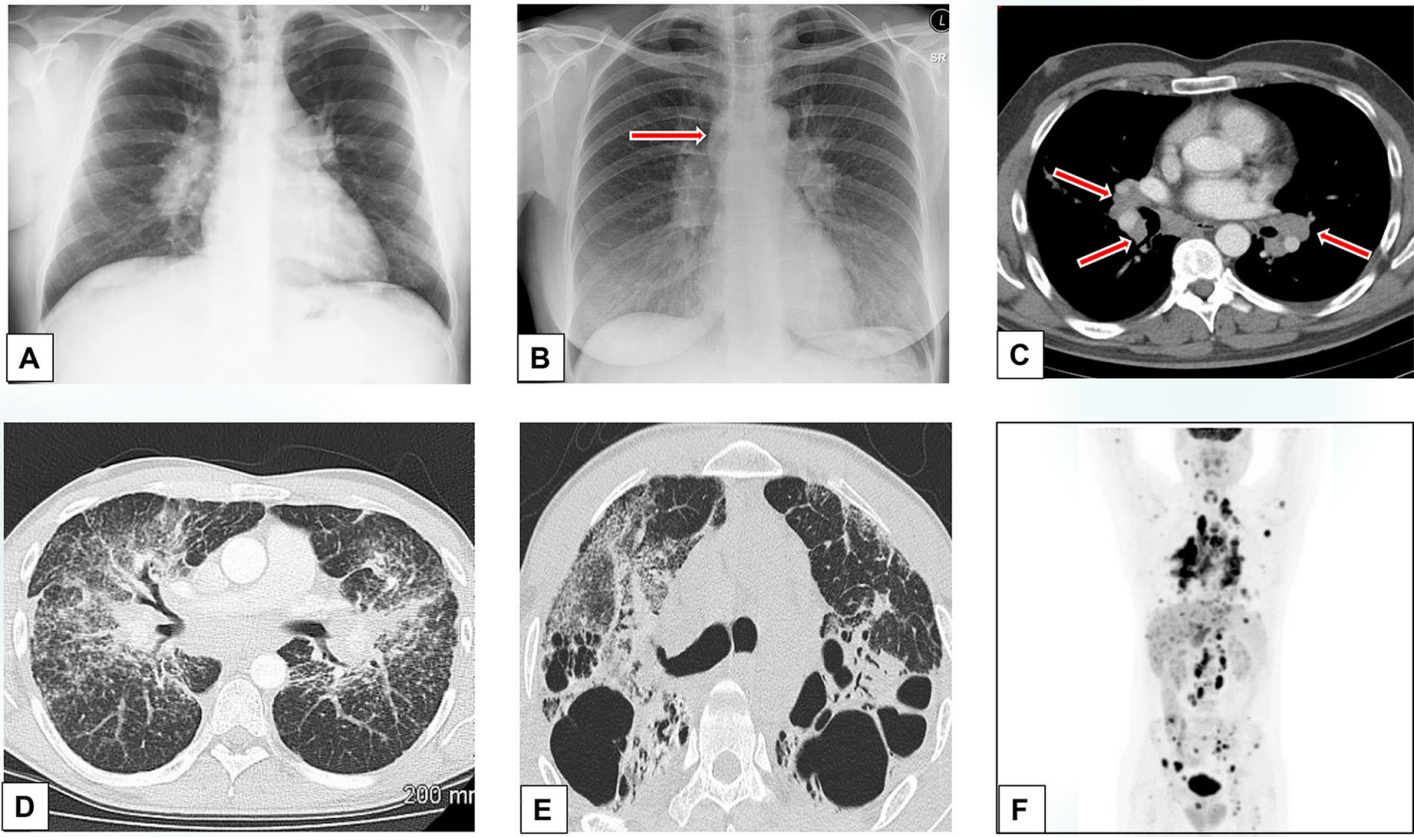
Radiographic patterns seen in the thorax of patients with systemic (including ophthalmic) sarcoidosis. a Bilateral hilar lymphadenopathy (BHL) with polycyclic outlines. b In another patient, BHL is associated with mediastinal enlargement along the right para-tracheal boundary (arrow). c Axial contrast computed tomography (CT) image shows typical BHL (arrows). Lymph nodes with a density lower than that of the vasa are disposed around the bronchi and pulmonary arteries. d Micronodules a few millimeters in diameter tend to form granulomatous conglomerates that surround the broncho-vascular peduncles. Lung involvement extends along the broncho-vascular axis, from the hila toward the periphery. Note the diffuse distribution in both lung fields of lung nodules with a typical peri-lymphatic distribution and thickening of the pleural surfaces. e Advanced-stage pulmonary sarcoidosis. Fibrosis is typically localized in the hilar and peri-hilar districts and in the upper lobes, with large cystic spaces. The hila are stretched upwards. f
